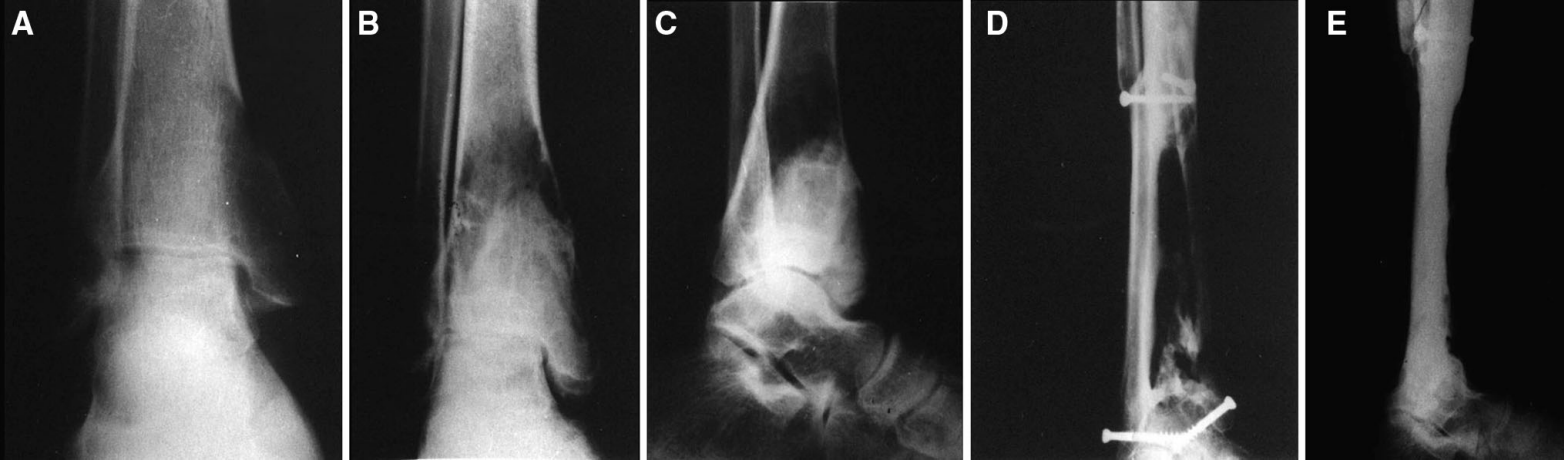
Radiograph of a 45-year-old male showing a giant cell tumor at the lower end of the tibia with a pathological fracture (a). Antero-posterior and lateral radiographs showing recurrence at 14 months after curettage and cementation (b, c). Follow-up radiographs at 6.5 years of the same patient treated with dual fibular grafts (d, e)
